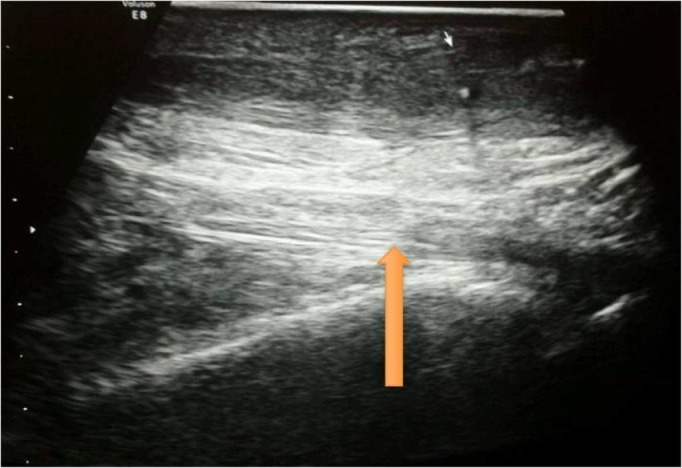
Ultrasonography of right Achilles tendon at nine months follow-up showing complete healing of the tendon.
